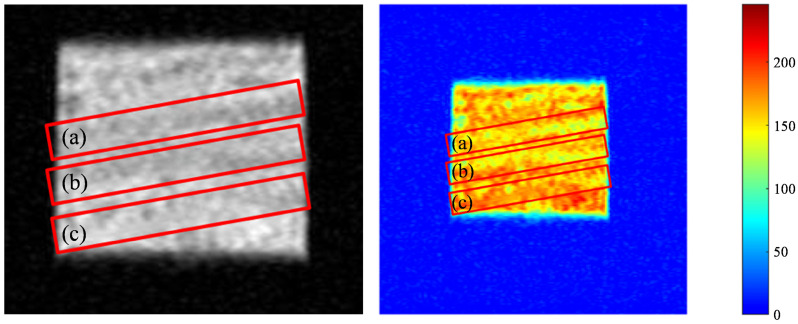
MRI scans of orthoclase cores: (a) first; (b) second; and (c) third layers.
